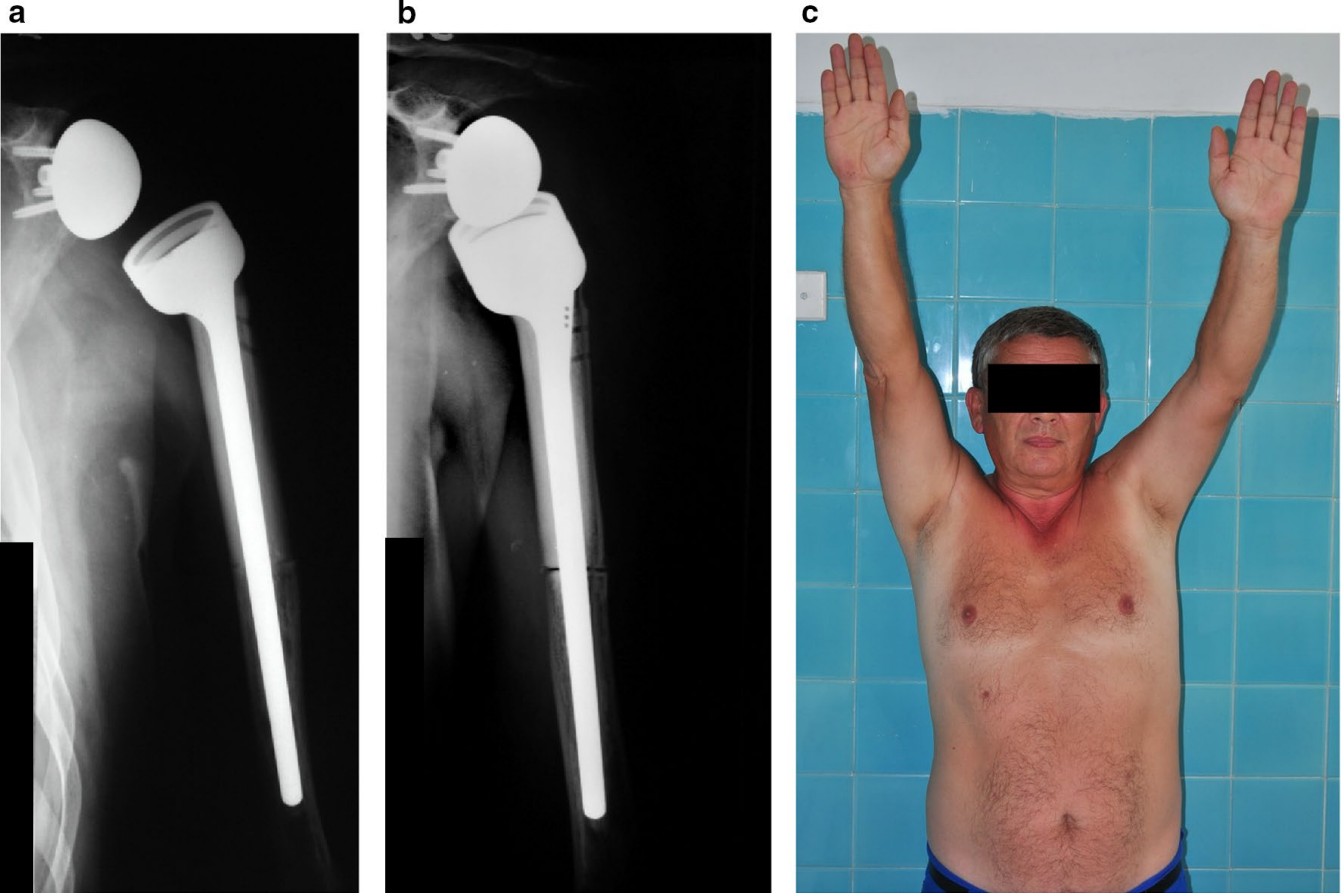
a Reconstruction of the left proximal humerus due to a chondrosarcoma using a reverse endoprosthesis-bone allograft. Patient developed instability in the shoulder joint in the post-operative period, so 4 months later the humeral component was augmented with a spacer. b Post-operative radiograph at 1 year following the primary procedure, and 8 months following spacer implantation. Deltoid muscle strength increased during the year, so consequentially instability decreased. c Good shoulder function and stable joint 1 year following surgery
